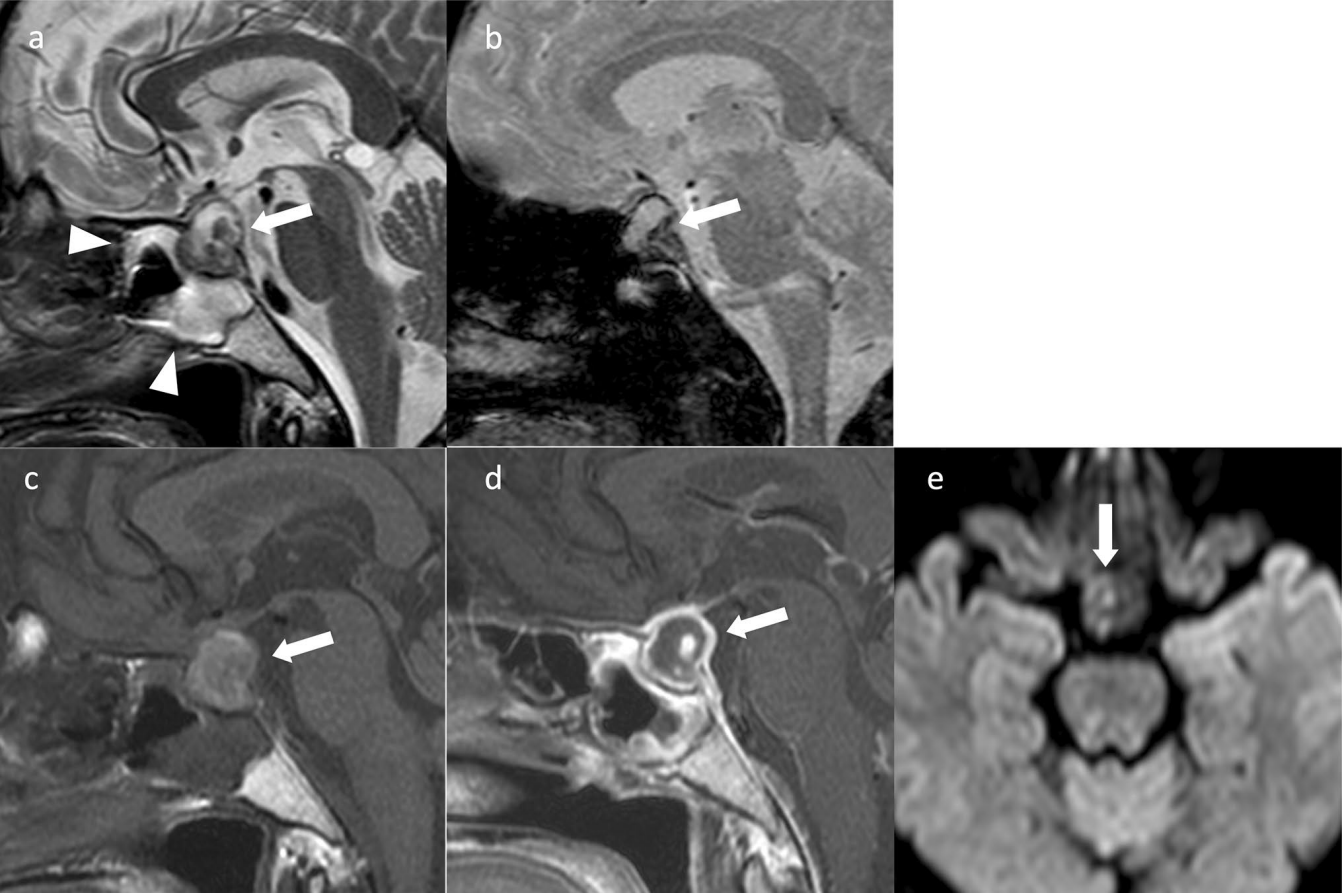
Pituitary apoplexy. A female patient in her 50 s, with headache, fever, and left ptosis. a Sagittal T2WI shows a mass in the sella turcica (arrow). The interior of the mass has a hyperintensity with some hypointensity regions. Mucosal thickening is observed in the sphenoid sinus (arrowhead). b Sagittal T2*WI reveals a hypointensity region in the mass, suggesting hemorrhage (arrow). c Sagittal T1WI shows a mildly hyperintensity mass (arrow). d Sagittal contrast-enhanced T1WI shows a ring-enhancing mass (arrow). e DWI shows iso-intensity (arrow). The patient underwent surgery via the transsphenoidal sinus approach, and hemorrhage and necrosis were observed
